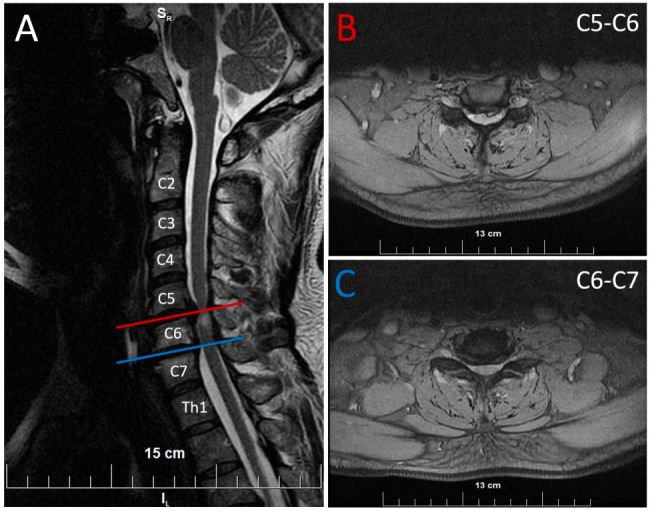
Magnetic resonance imaging (MRI) of the cervical spine. (A): T2-weighted sagittal MRI showing spinal cord, bulging of the intervertebral discs at all cervical levels and disc herniation at levels C5-C7 with myelopathy. Horizontal lines indicate the corresponding levels in the axial views. (B): T2-weighted axial MRI at the level of C5-C6 — paramedian disc herniation to the left with compression of the dural sac and the spinal cord with myelopathy. (C): T2-weighted axial MRI at the level of C6-C7—median disc herniation with compression of the dural sac and the spinal cord with myelopathy.
